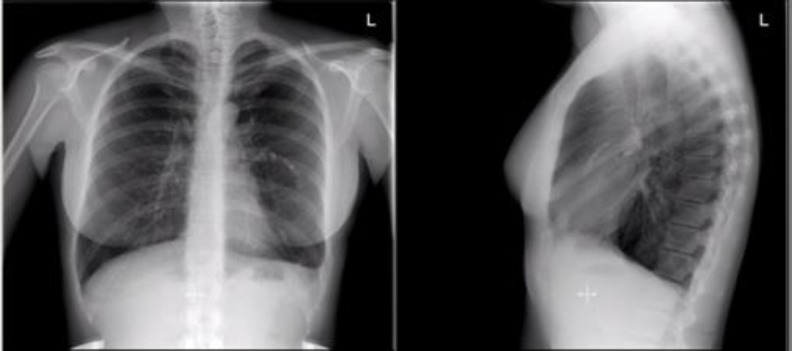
Postembolization chest x-ray showing six embolization coils with complete resolution of pleural effusion
